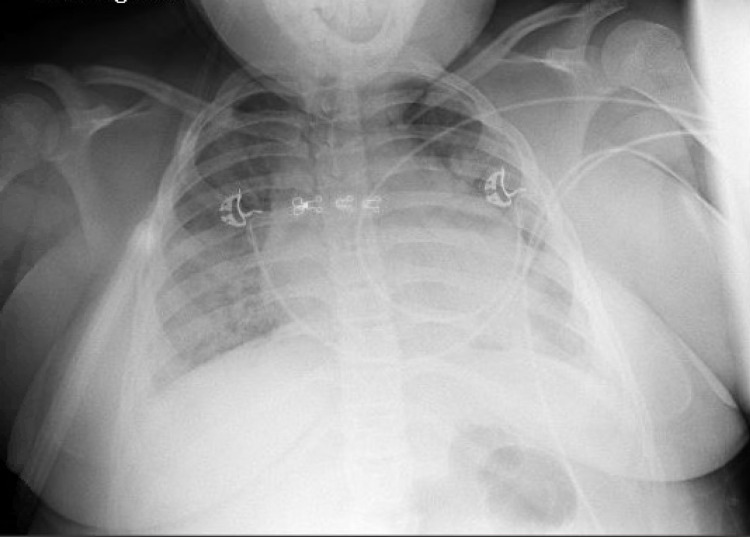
Chest radiograph showing diffuse bilateral interstitial opacities.
